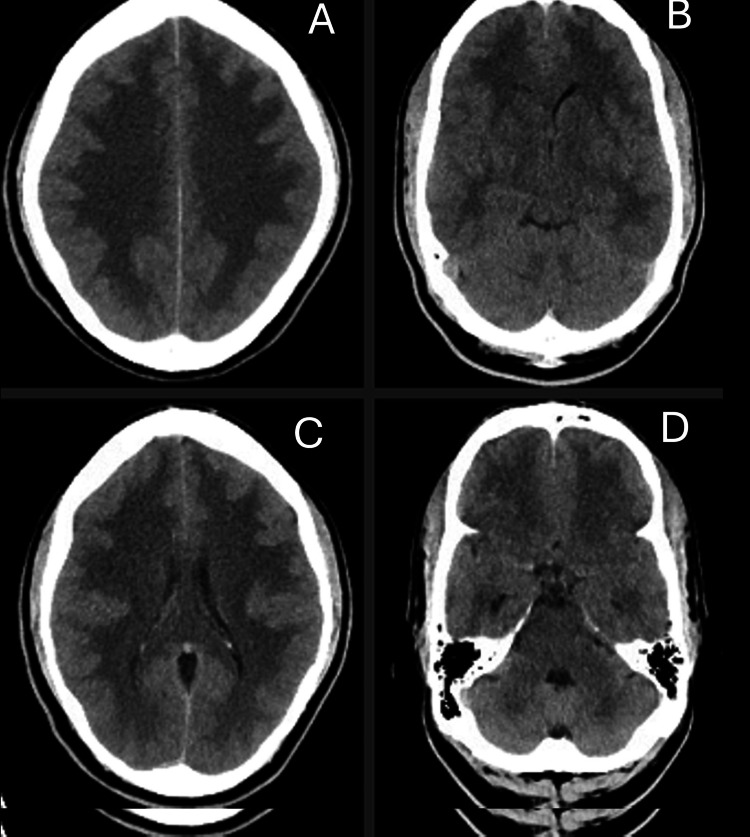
Initial CT scan images of the head Initial CT scan of the head (at the time of presentation) demonstrating diffuse white matter hypoattenuation predominantly involving bilateral frontoparietal (A) and temporal lobes (B) and partial involvement of the occipital lobes (C) and brain stem and cerebellum (D).
